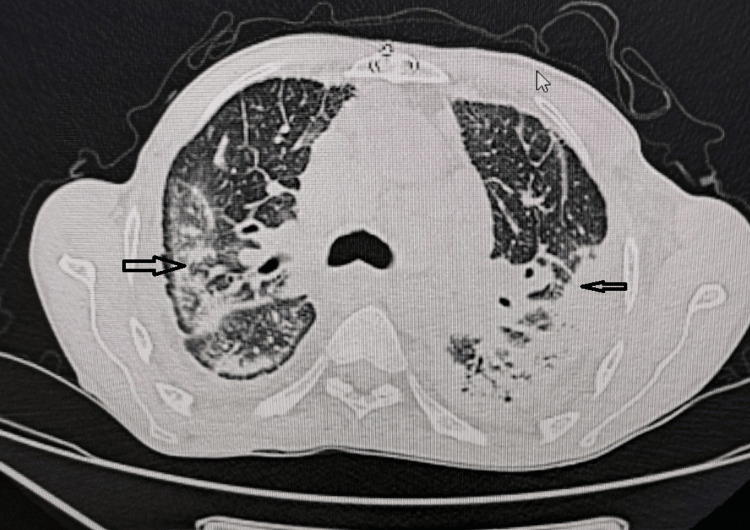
Case 3: Chest CT in axial section, parenchymal window, showing extensive pneumonic consolidations in a patient with common variable immunodeficiency, associated with bilateral bronchiectasis.
